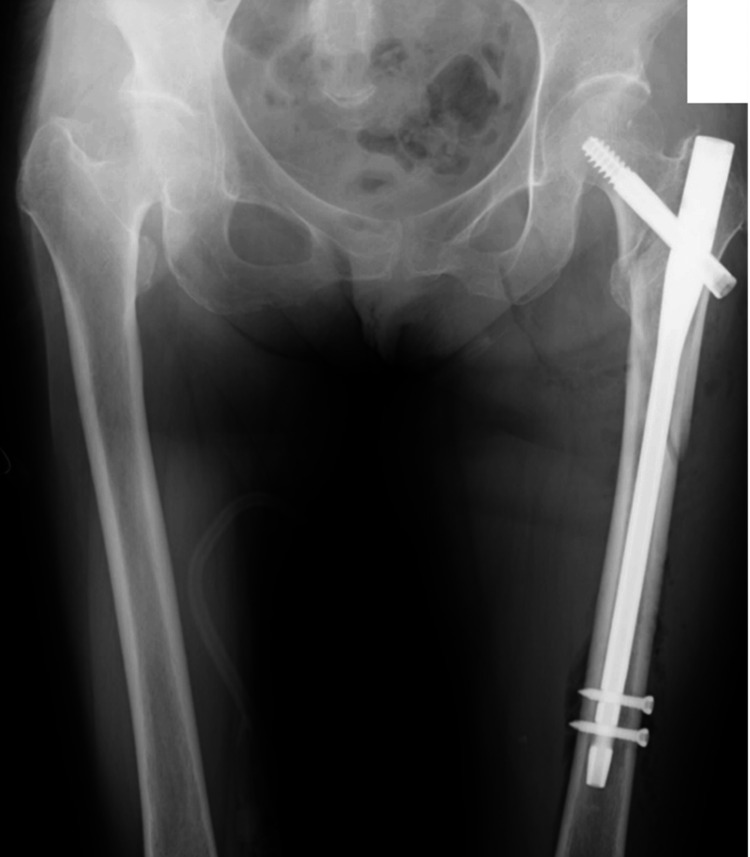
Postoperative X-ray The surgical procedure involved an IPT-EF nail (125°×260mmφ10mm; HOMS Engineering Inc., Nagano, Japan), and the NESPLON Cable System (Alfresa Pharma Corporation, Osaka, Japan) was used for subtrochanteric fixation.
